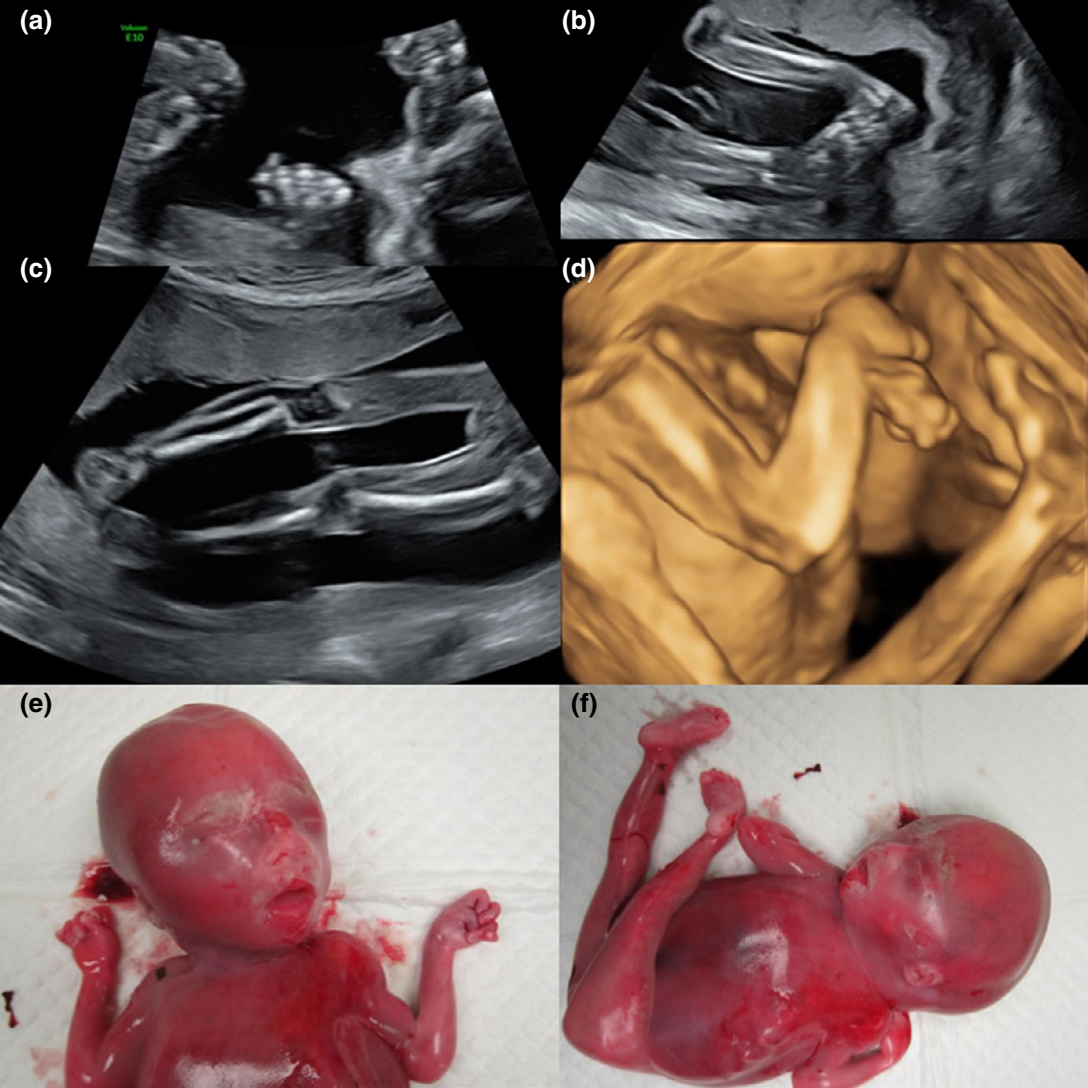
Ultrasound images and clinical photos from case 5. Ultrasound images show closed hand (a), bilateral talipes (b), extended legs (c), and flexed arms and wrists (d). Clinical photos of the abortus show bilateral flexed elbows, closed hands (e), and extended legs with bilateral clubfeet (f)
